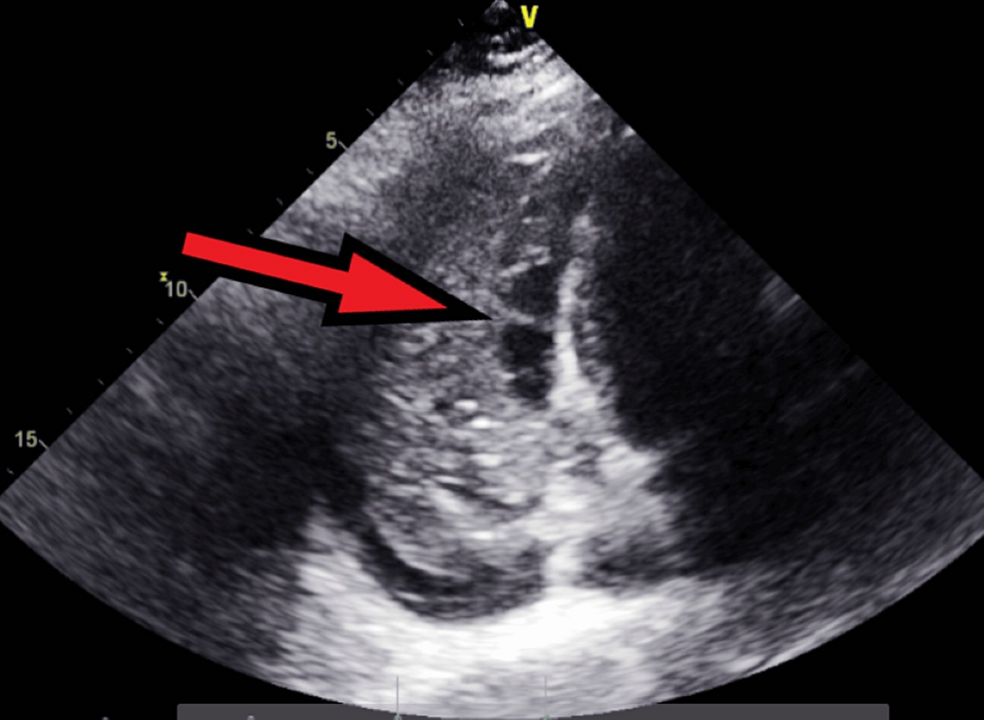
Transthoracic echocardiography post-pericardiocentesis - image 3 Transthoracic echocardiography post-pericardiocentesis; apical 4-chamber view showing multiple loculations (red arrow) with some remnant pericardial fluid collections
